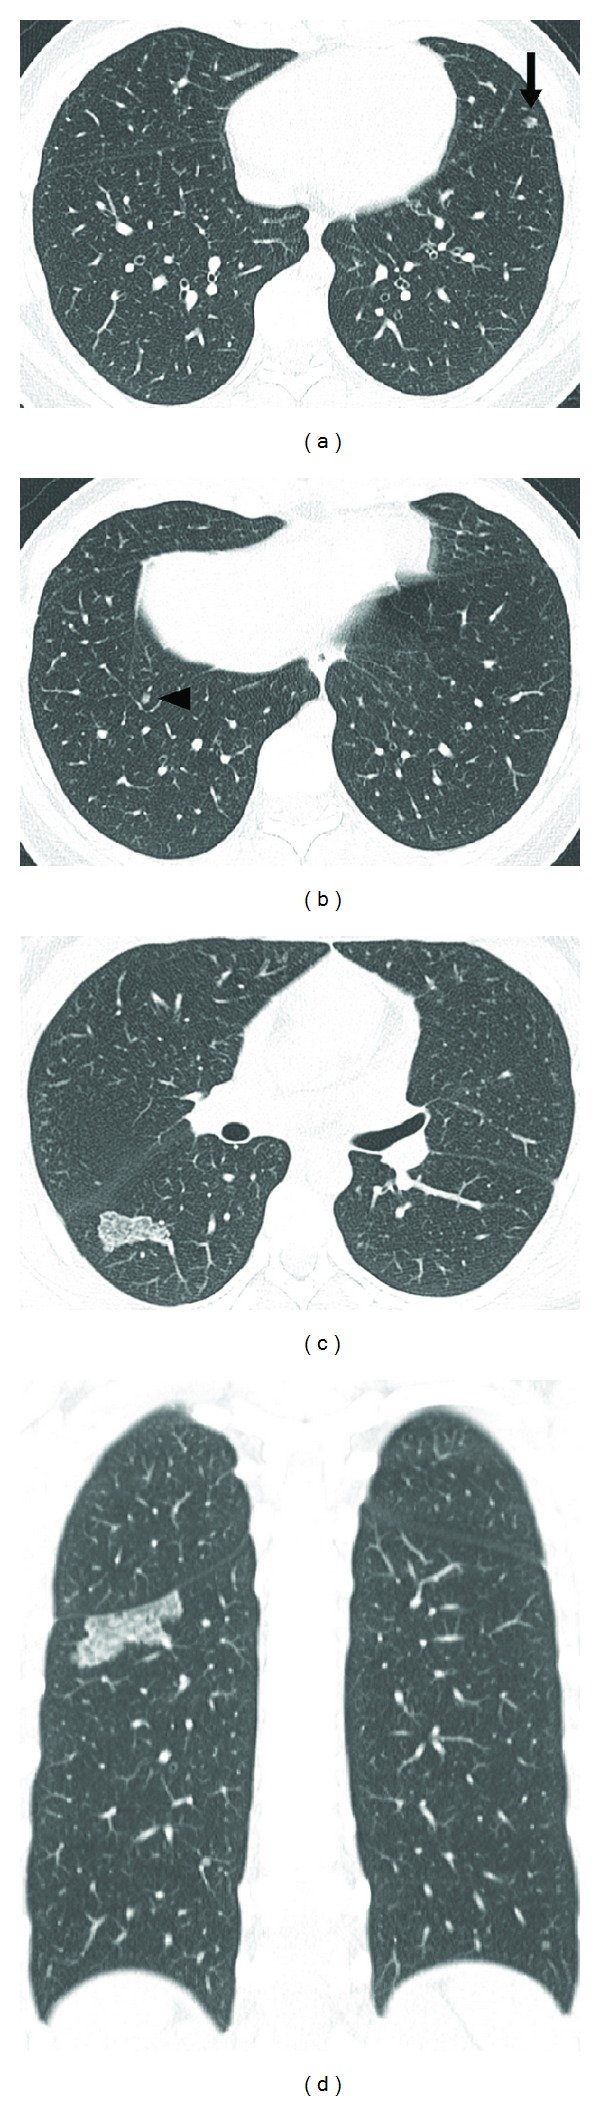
Chest CT findings. (a), (b) Axial CT images (1.5 mm thickness) of the chest show small nodules with ground-glass opacity in the lingular segment of the left upper lobe ((a), arrow) and the lateral segment of the right lower lobe ((b), arrowhead). (c) Axial CT image (1.5 mm thickness) shows ground-glass opacity with 30 mm diameter surrounded by dense linear consolidation (reversed halo sign) in the superior segment of the right lower lobe. (d) Coronal reconstructed image (3 mm thickness) also shows revered halo sign in the superior segment of the right lower lobe.
